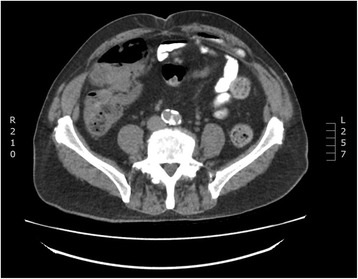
Computed tomography scan showing posterior rectus sheath hernia of a preperitoneal subtype
